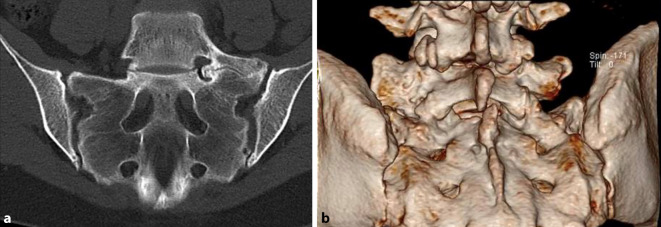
CT-Darstellung (a) einer lumbosakralen Übergangsanomalie Typ Castellvi IIA mit 3‑D-Rekonstruktion (b)
